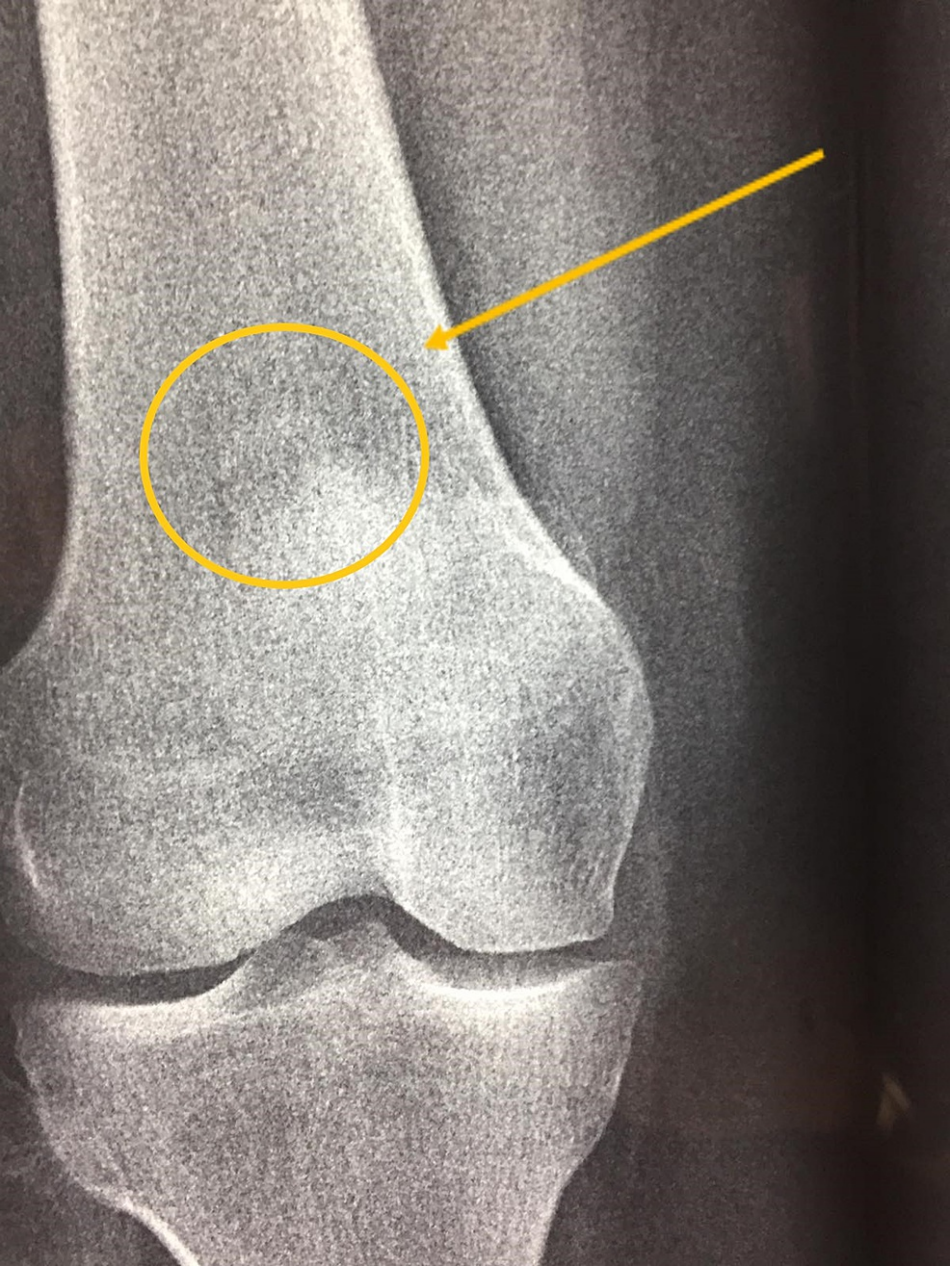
Anteroposterior radiograph of the patient's knee showing the bone lesion (yellow circle and arrow).
